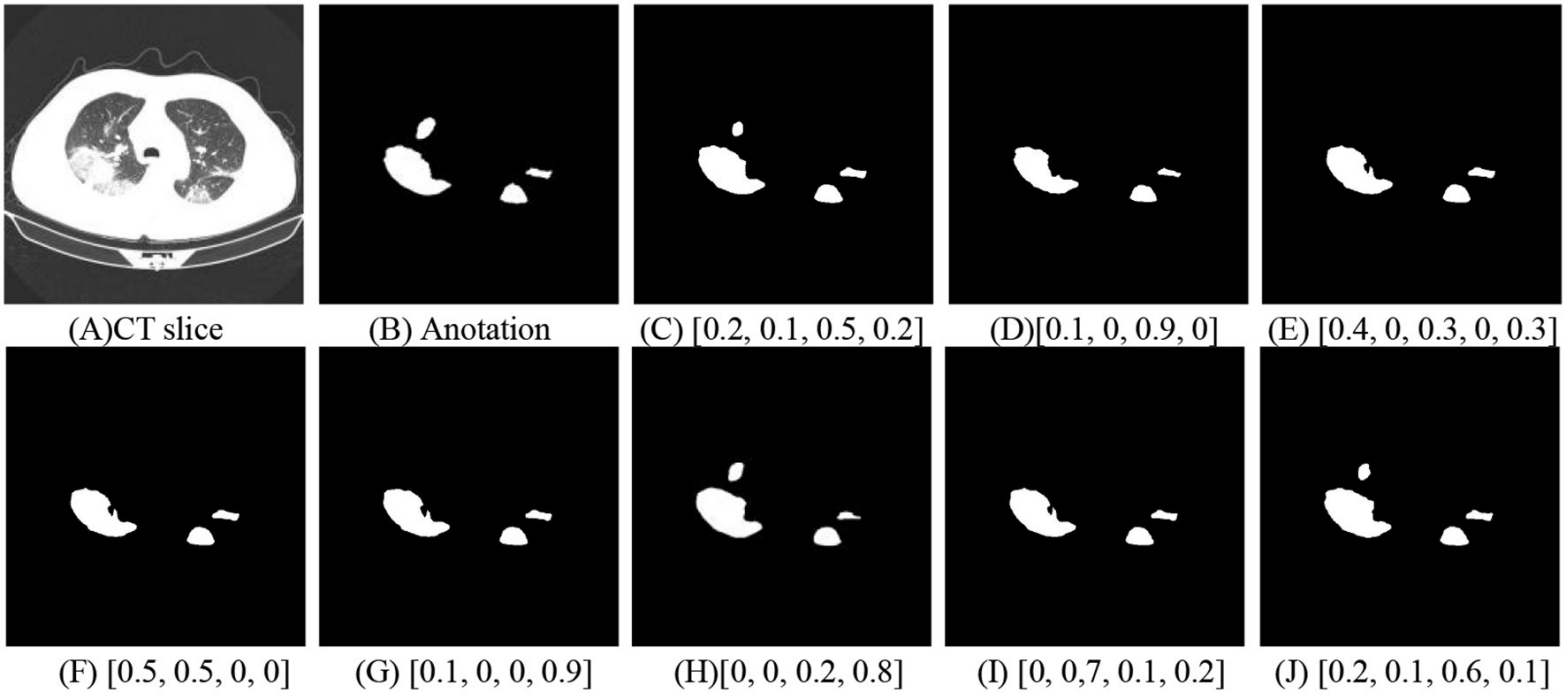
COVID-19 lesion segmentation with different weighting parameters. (A) CT slice. (B) Anotation. (C) [0.2, 0.1, 0.5, 0.2]. (D) [0.1, 0, 0.9, 0]. (E) [0.4, 0, 0.3, 0, 0.3]. (F) [0.5, 0.5, 0, 0]. (G) [0.1, 0, 0, 0.9]. (H) [0, 0, 0.2, 0.8]. (I) [0, 0.7, 0.1, 0.2]. (J) [0.2, 0.1, 0.6, 0.1].
